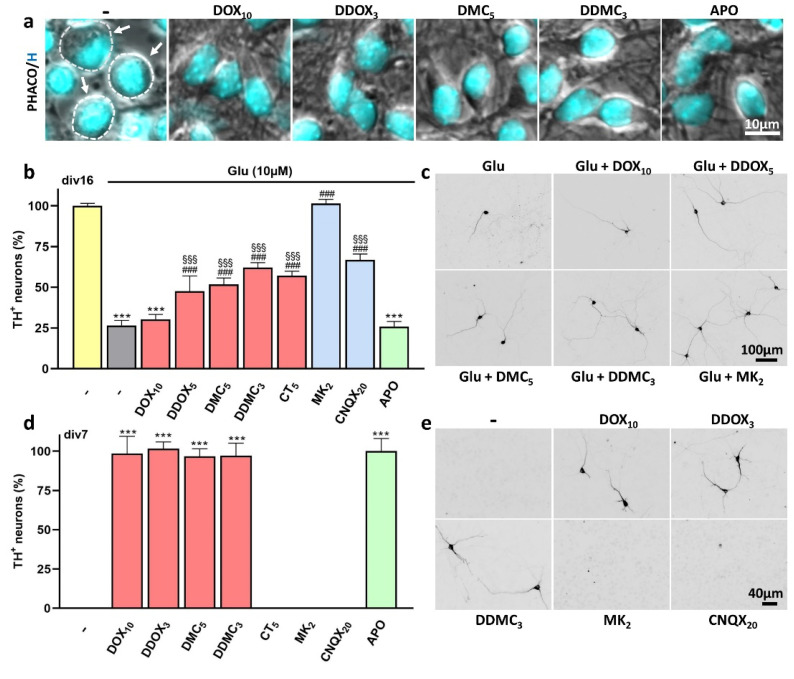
TC-mediated neuroprotection of DA neurons against iron-mediated neurodegeneration is not explained by blockade of glutamate excitotoxicity. (a) Phase contrast (Phaco) images of div2–3 cultures showing that protective TC derivatives and APO prevent the swelling of neuronal cell bodies that is prominent when iron-dependent insults culminate in NT cultures. White arrows point to swollen neuronal cell bodies whose boundaries are delineated with a dashed line. Note that nuclear integrity (H-33342; blue signal) is preserved not only in the presence, but also in the absence, of protective treatments at this stage of neurodegeneration. (b) Survival of div16 DA neurons exposed 2 d before to Glu (10 µM) in the presence or absence of DOX (10 µM), DDOX (5 µM), DMC (5 µM), DDMC (3 µM), CT (5 µM), APO (100 µg/mL), MK-801 (MK, 2µM), or CNQX (20 µM). Data expressed in % of NT cultures are means ± SEM (6–15). * *p* < 0.001 vs. NT; ^###^
